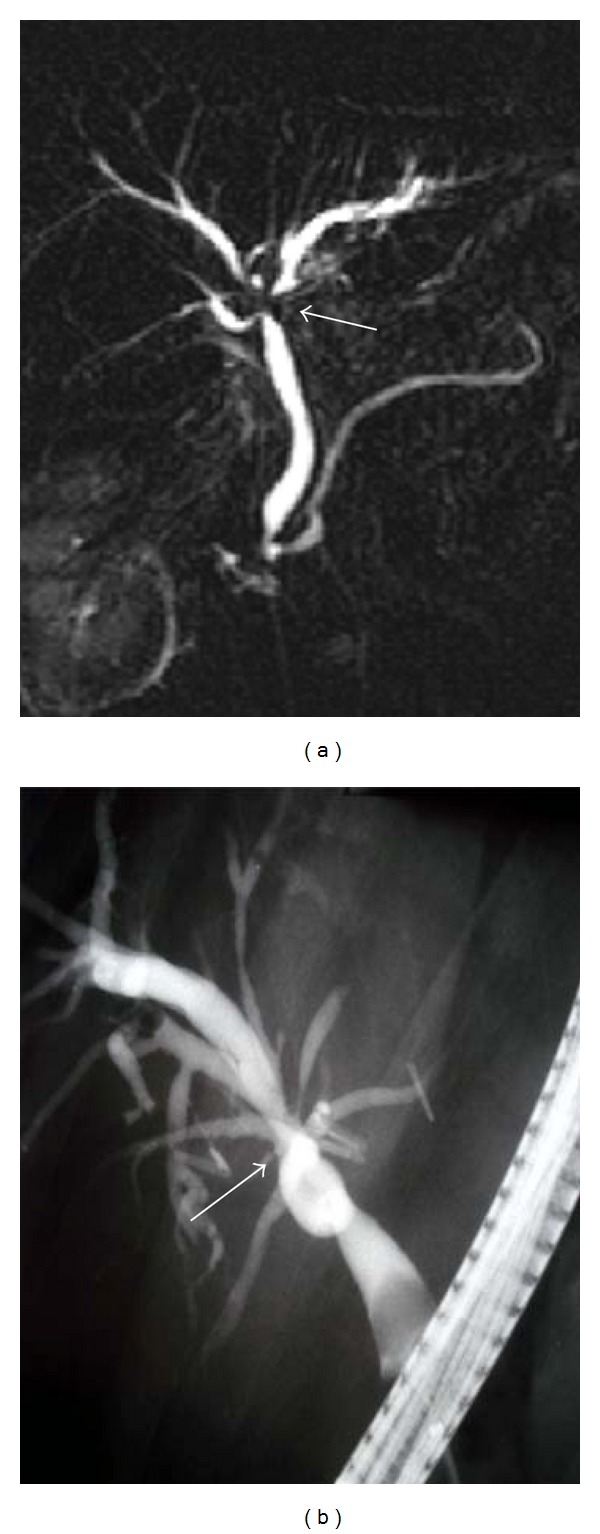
In a case who had undergone laparoscopic cholecystectomy operation 3 years ago, MRCP image demonstrates (a) stricture at hilar level (arrows) and mild dilatation of left main hepatic duct. Although this case had received false positive diagnosis as malignant stricture with MRI and MRCP findings, during ERCP, benign stricture developed secondary to cholecystectomy was diagnosed (arrows). Balloon dilatation and stent replacement was performed during ERCP procedure, and the result of biopsy was benign.
